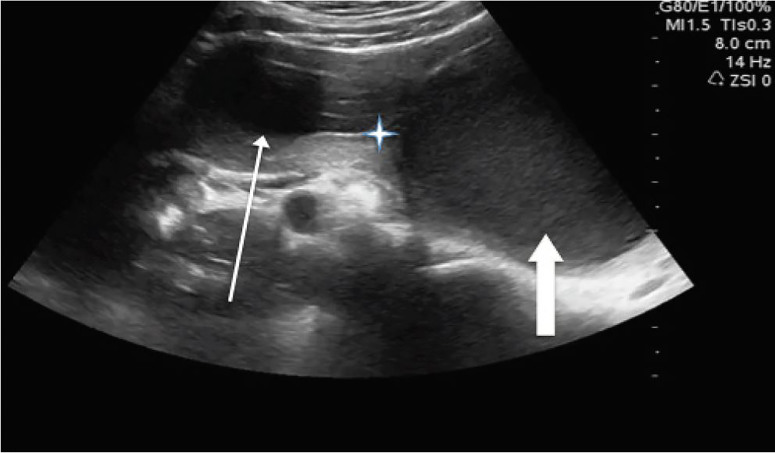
Phased-array, transverse ultrasound scan in the right adnexal region demonstrating a blind hemivagina (thin arrow) and the uterus (thick arrow). Both structures are filled with hypoechoic material and separated by a hyperechoic wall (star).
